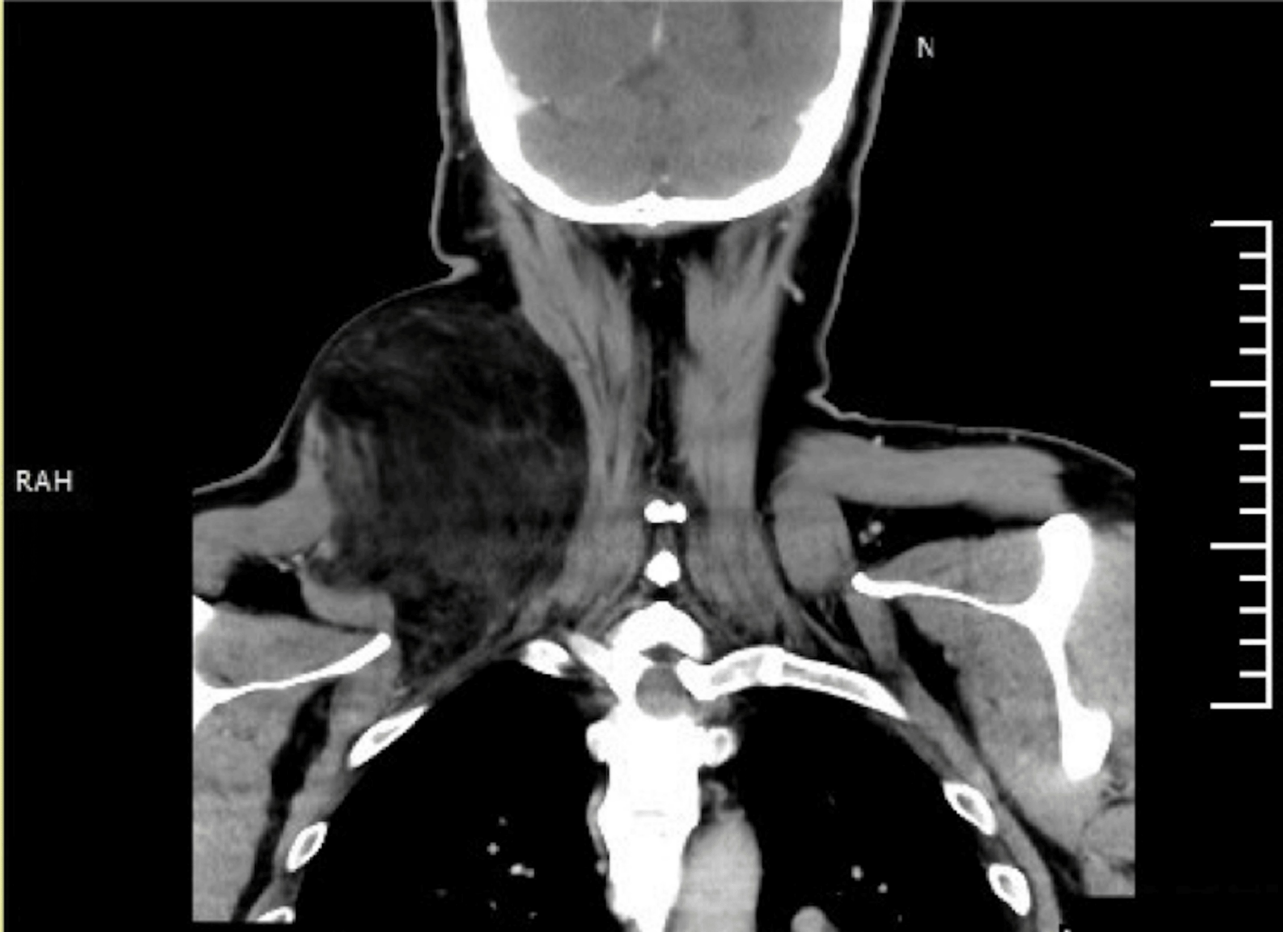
A coronal view of the neck CT scan shows a right-side mass compressing the surrounding muscular structures. The mass demonstrates multiple septations, small soft tissue nodules, fluid density areas, and curvilinear foci of calcifications, with no evidence of soft tissue enhancement.
